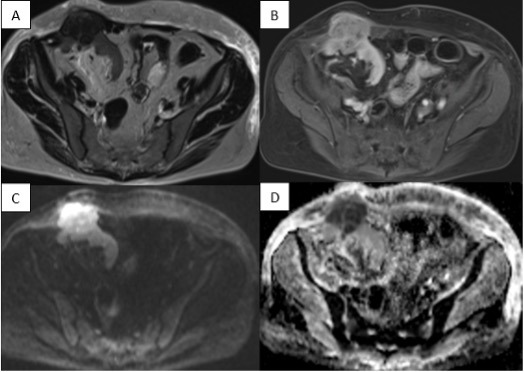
MRI of the transplanted kidney showed a T2-hypointense lesion (A) with enhancement after gadolinium contrast administration on the T1 fat-saturated sequence (B) and restricted diffusion (C, D).
